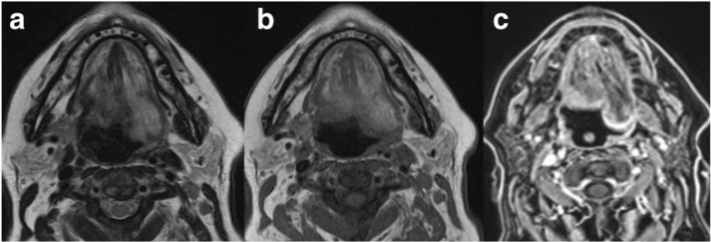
Patient with hypoglossal nerve palsy on the left. The T2-weighted MR image a the T1-weighted MR image b and the T1-weighted MRI image with fat saturation after injection of gadolinium c shows the fatty changes of the left side of the tongue and a dorsal bulge due to loss of muscle tone. This should not be interpreted as a tumour on the left side
